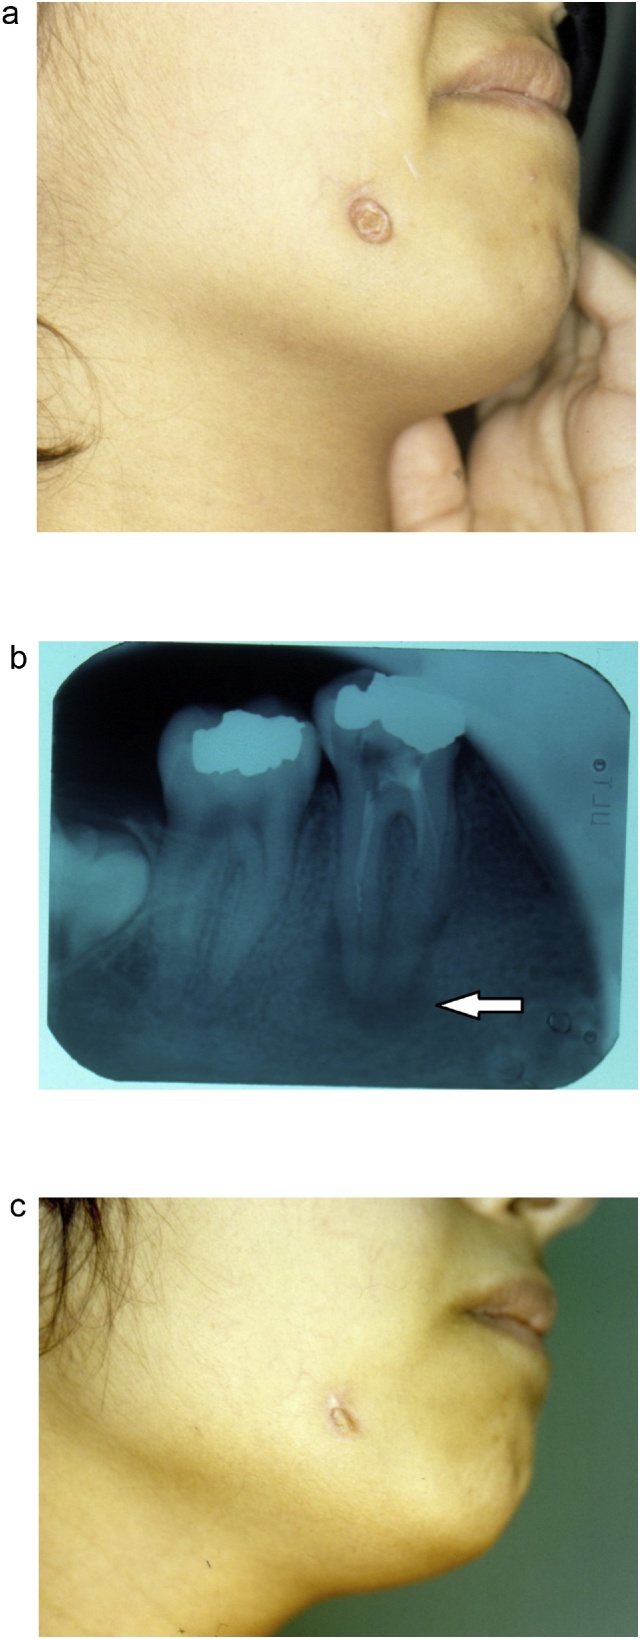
a) A nodulo-ulcerative lesion along the mandibular border. There was an adjacent teeth caries. b) Dental x-rays showing the radiolucency around the chronically infected tooth root (arrow). c) Resolution of the ulcer following dental extraction.
